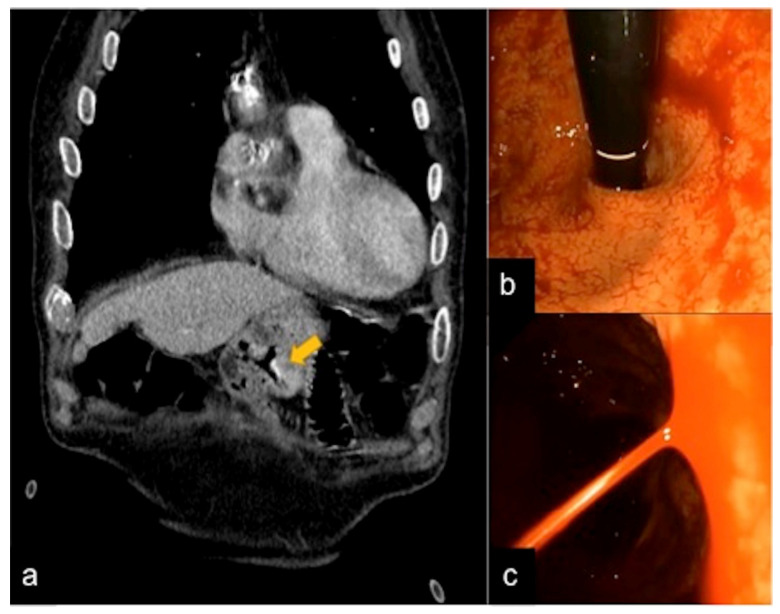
CTA multiphasic study of a gastric Dieulafoy lesion. In the arterial phase (a) and relative endoscopic evaluation (b,c), a vascular sub-mucosal lesion is identified (a, arrow).
